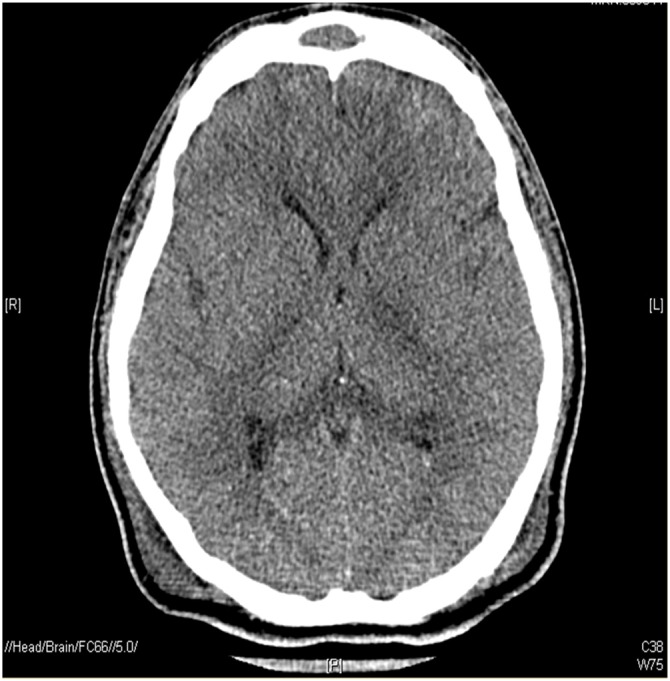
Patient computed tomography scan recorded 43 days post-trauma.
